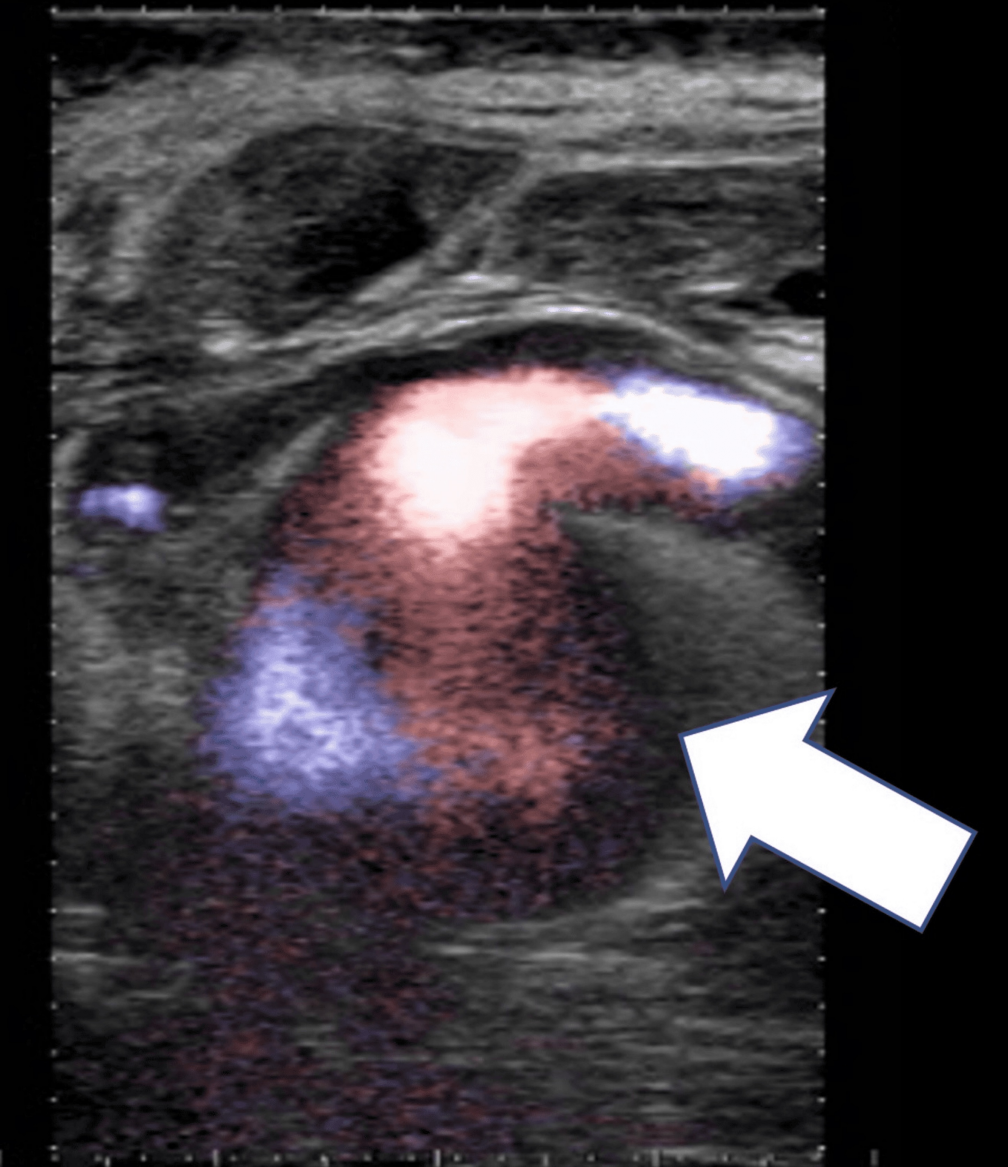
Point-of-care ultrasound showing the yin-yang sign (white arrow).
